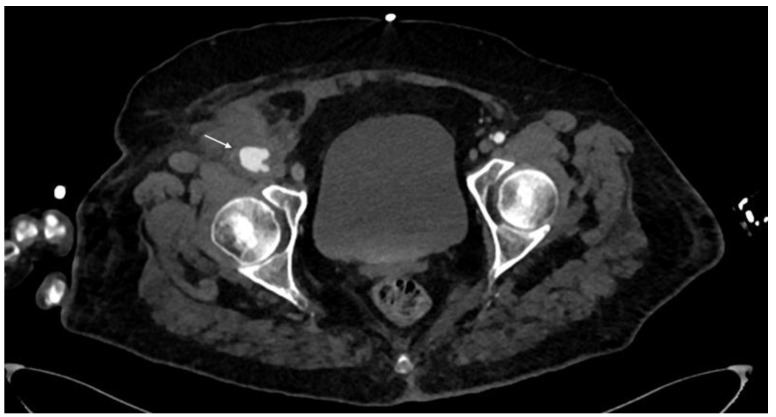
CTA, axial planes. Right common femoral artery pseudoaneurysm can be noted (arrow). It appears as an outpouching sac with a round margin in continuity with the arterial-adjacent lumen. In this case, imminent signs of rupture of the pseudoaneurysm can be seen as irregular and lobulated margins and the adjacent hematoma.
